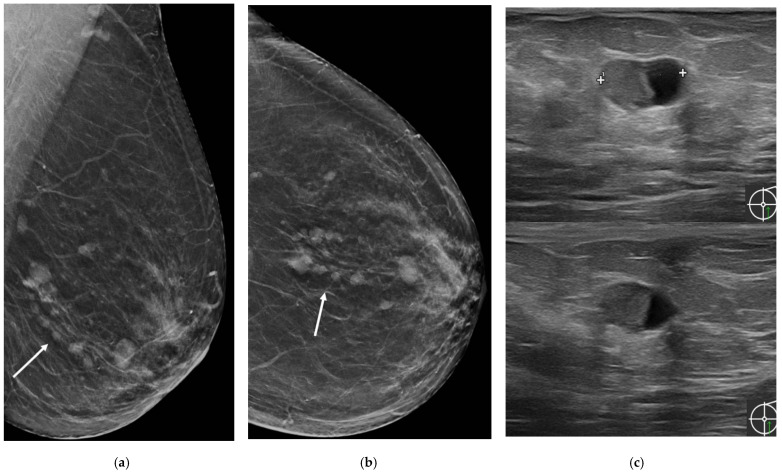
A 55-year-old asymptomatic woman. (a) Medio-lateral view and (b) cranio-caudal view of screening mammography demonstrated small round or oval opacities in the lower-outer quadrant of the left breast (arrows); (c) Ultrasound examination showed multiple intracystic masses.
